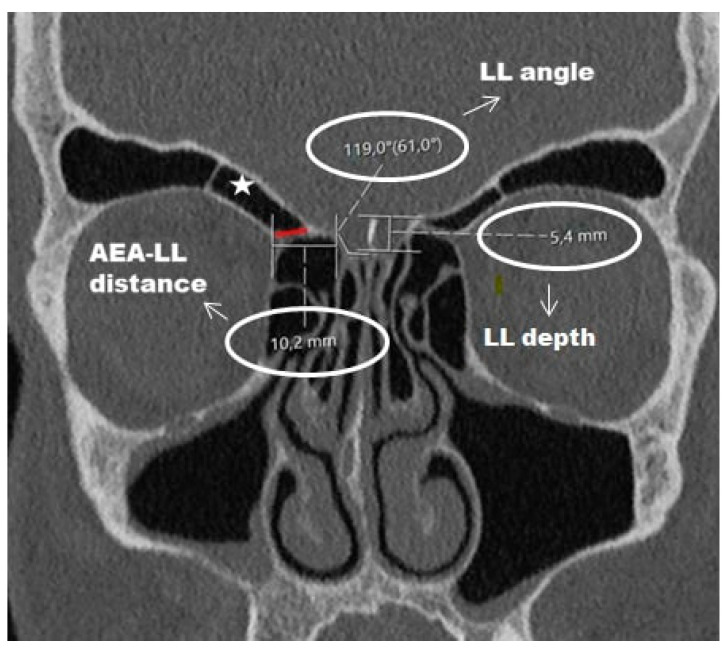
Representative coronal CT image demonstrating key morphometric measurements of the ethmoid roof and lateral lamella.
